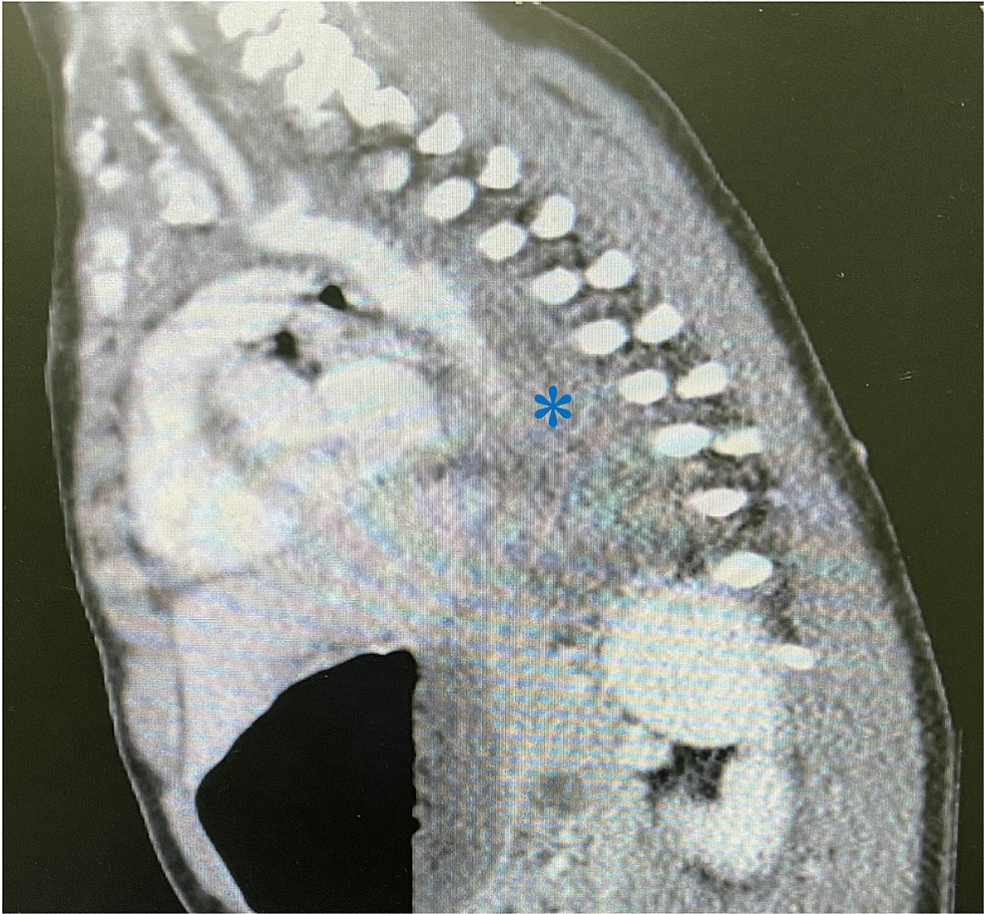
CT sagittal view showing grade III aortic pseudoaneurysm (asterisk)
